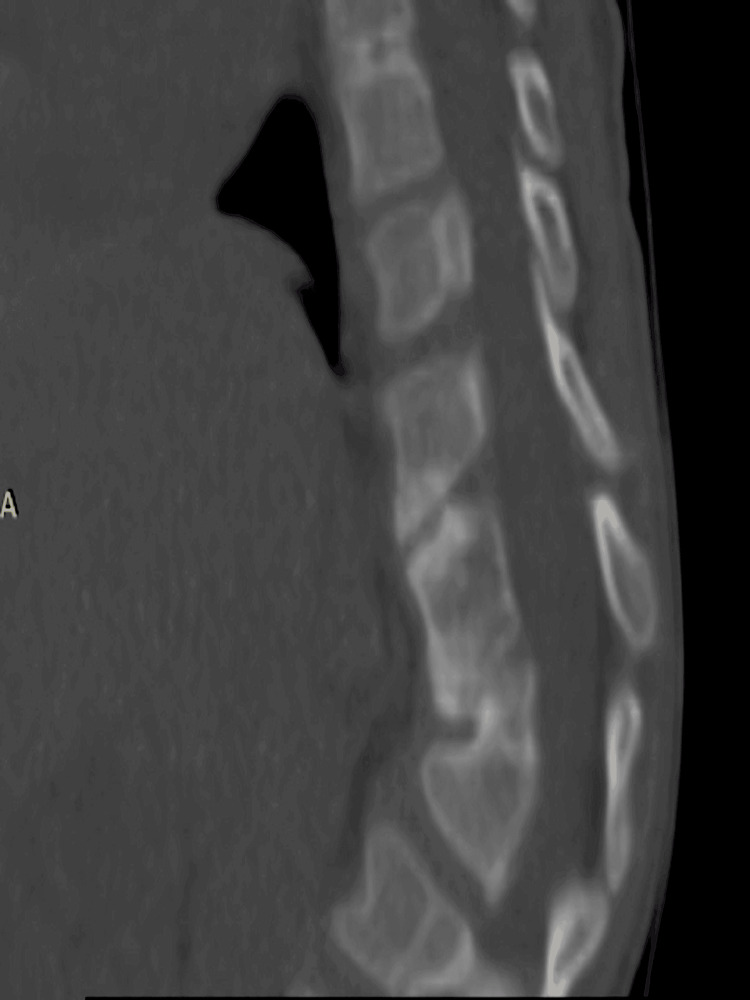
A pre-operative CT of the thoracolumbar spine depicts the kyphosis level and the hemivertebrae.
